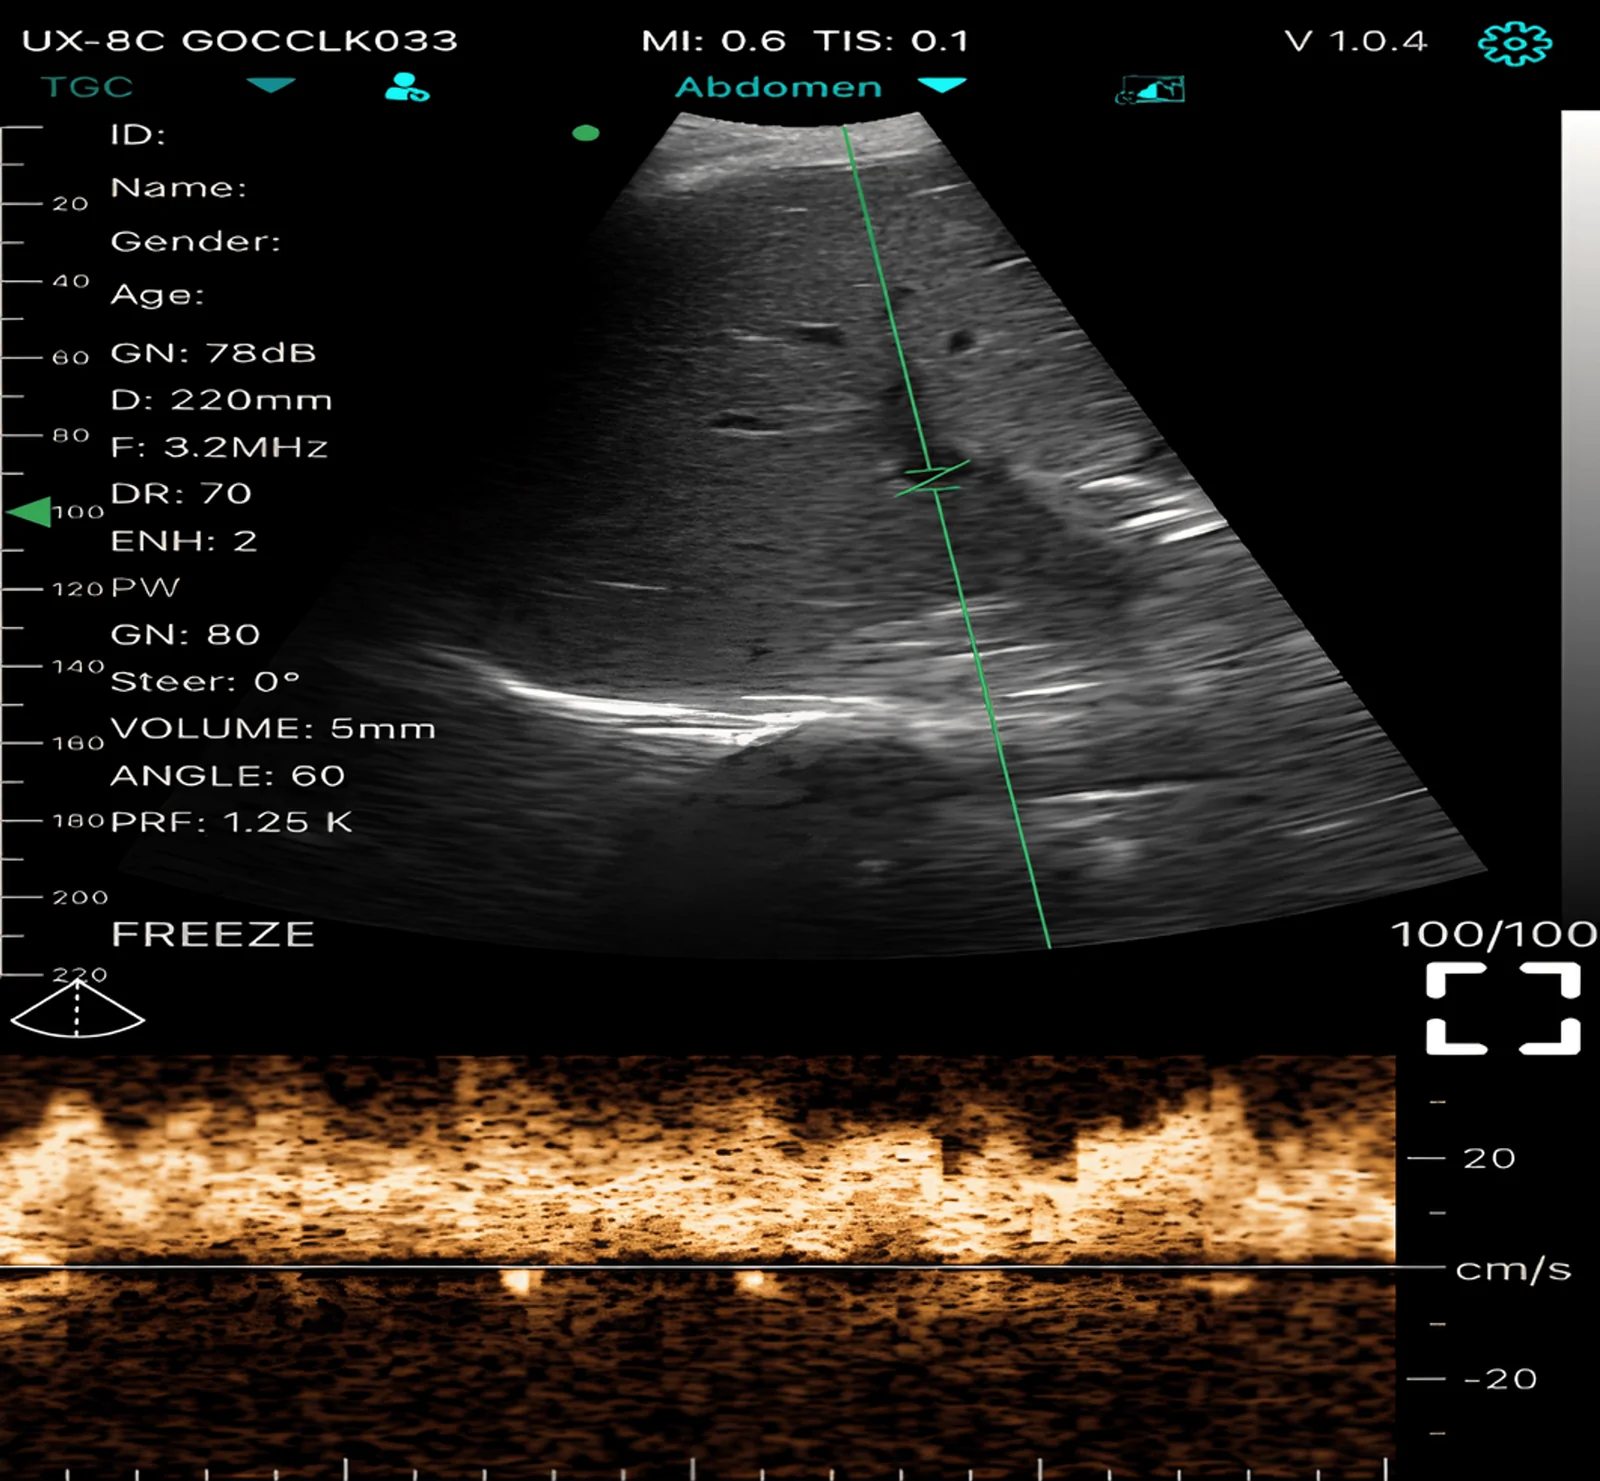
Assessment of the portal vein waveform by wireless handheld ultrasound
